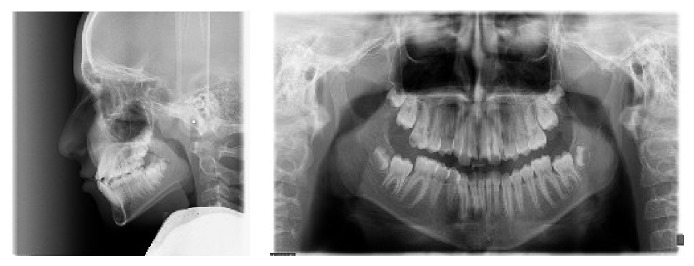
Pretreatment lateral cephalometry and panoramic radiographs.
